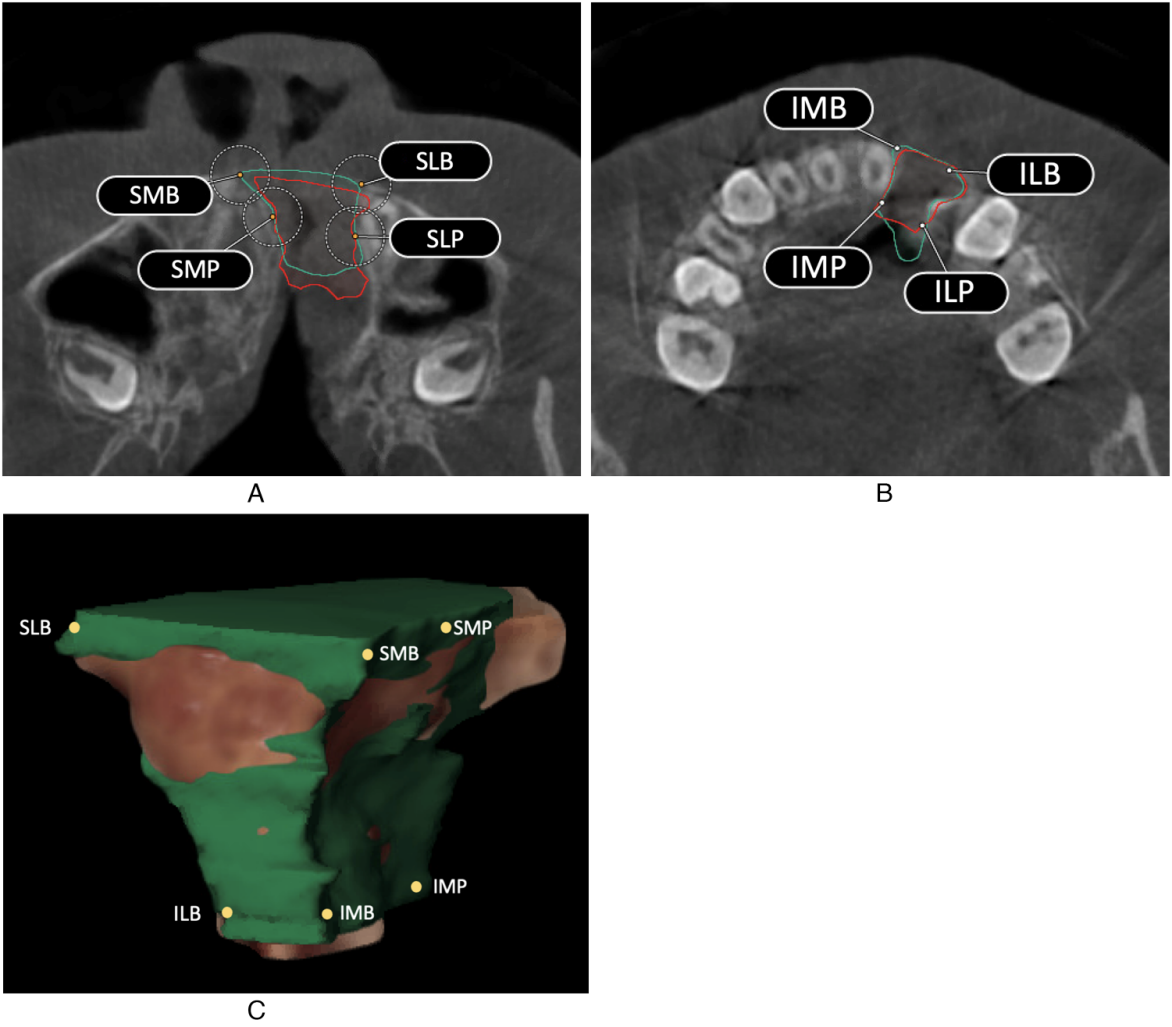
Display of the landmarks in the alveolar cleft. (A) Axial CBCT slice of most cranial
